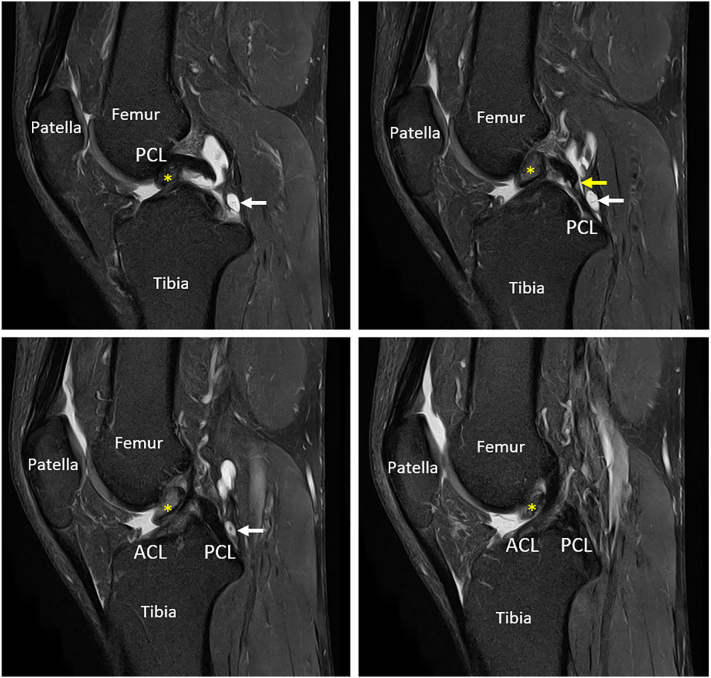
The tumor on sagittal plane MRI of the patient's left knee. Yellow asterisk: tumor; yellow arrow: neglected injury of PCL; white arrow: cyst of PCL; ACL: anterior cruciate ligament; PCL: posterior cruciate ligament.
